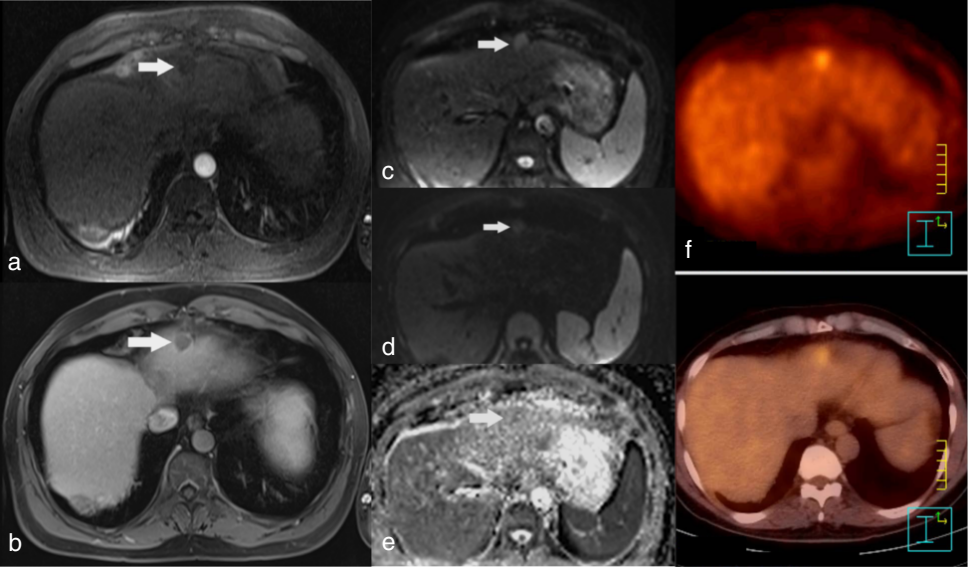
Poorly differentiated HCC in a patient with left lobe HCC, showing no restricted ADC on DWI-MRI and increased uptake on FDG-PET/CT. Dynamic 3D gradient echo T1 WI shows a non enhancing nodule on arterial phase (a, *arrow*), remaining hypo intense on portal phase (b, *arrow*) but associated to a thin peripheral enhanced rim. The nodule shows high signal intensity on DWI image with b = 0 s/mm^2^ (c, *arrow*) decreasing on DWI b = 800 s/mm^2^ images (d, *arrow*), although its signal intensity remained higher than the liver. The tumour ADC was 1.38 × 10^−3^ mm^2^/s (e, *arrow*), similar to that of the adjacent liver. The lesion proved positive on FDG-PET/CT (f)
